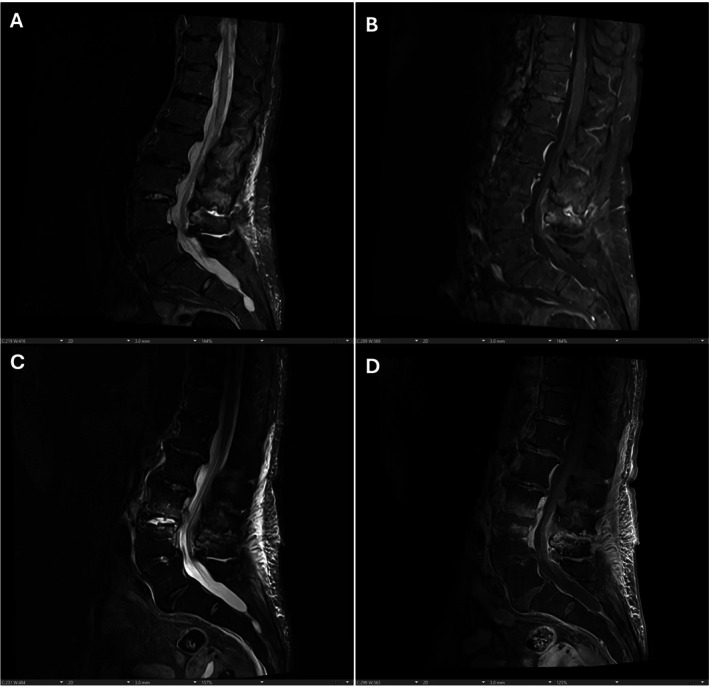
Sagittal MRI images of the lumbar spine comparing initial imaging (A and B) and repeat imaging approximately 1 month later (C and D), highlighting interval progression with increased T2‐weighted hyperintensity, greater enhancement, and worsening adjacent vertebral endplate edema at L3–L4, consistent with evolving discitis/osteomyelitis.
